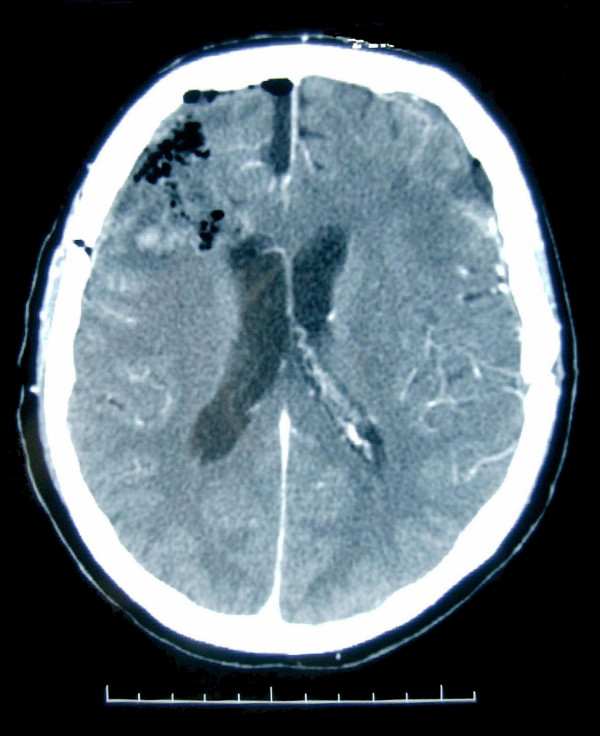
Brain Computed Tomography performed 24 h after second surgical intervention.
